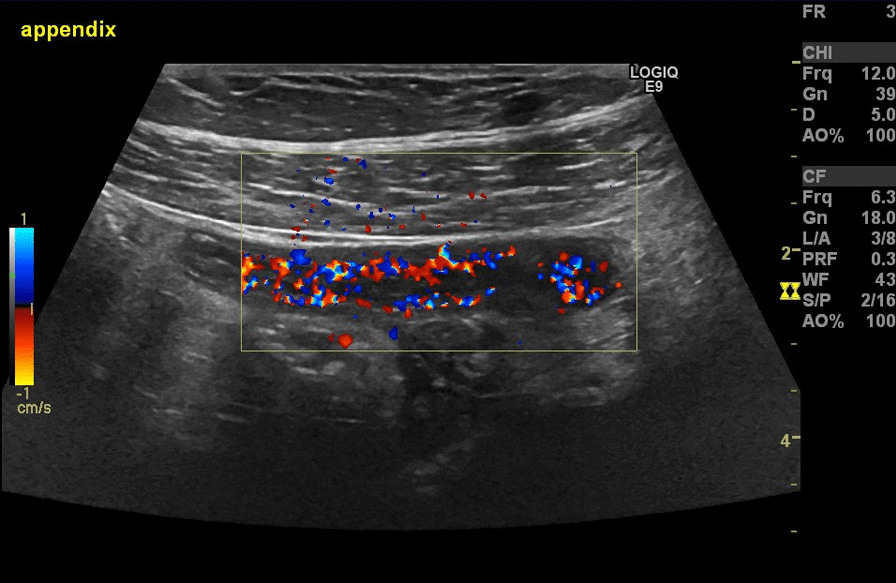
Patient 1. Ultrasound of lower right quadrant. Ultrasound of the right lower quadrant (area of maximum tenderness) displaying layering and thickening (10 mm) of the distal appendix with increased vascularity and moderate periappendicular edema. No abscess or signs of perforation
